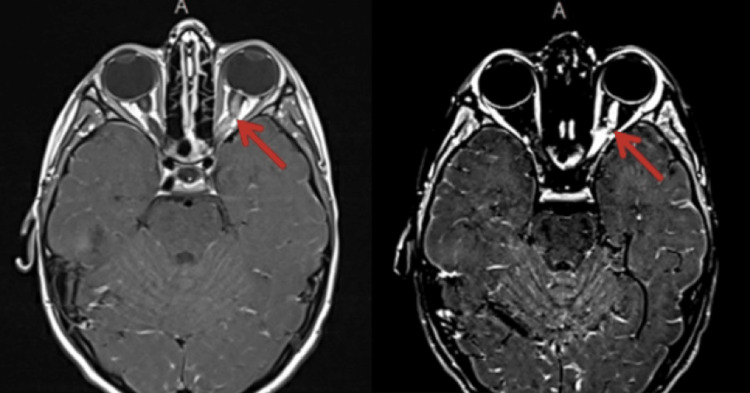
MRI of the brain showing left optic nerve enhancement during the first episode; MOG serology was negative at this time. MOG, myelin oligodendrocyte glycoprotein
